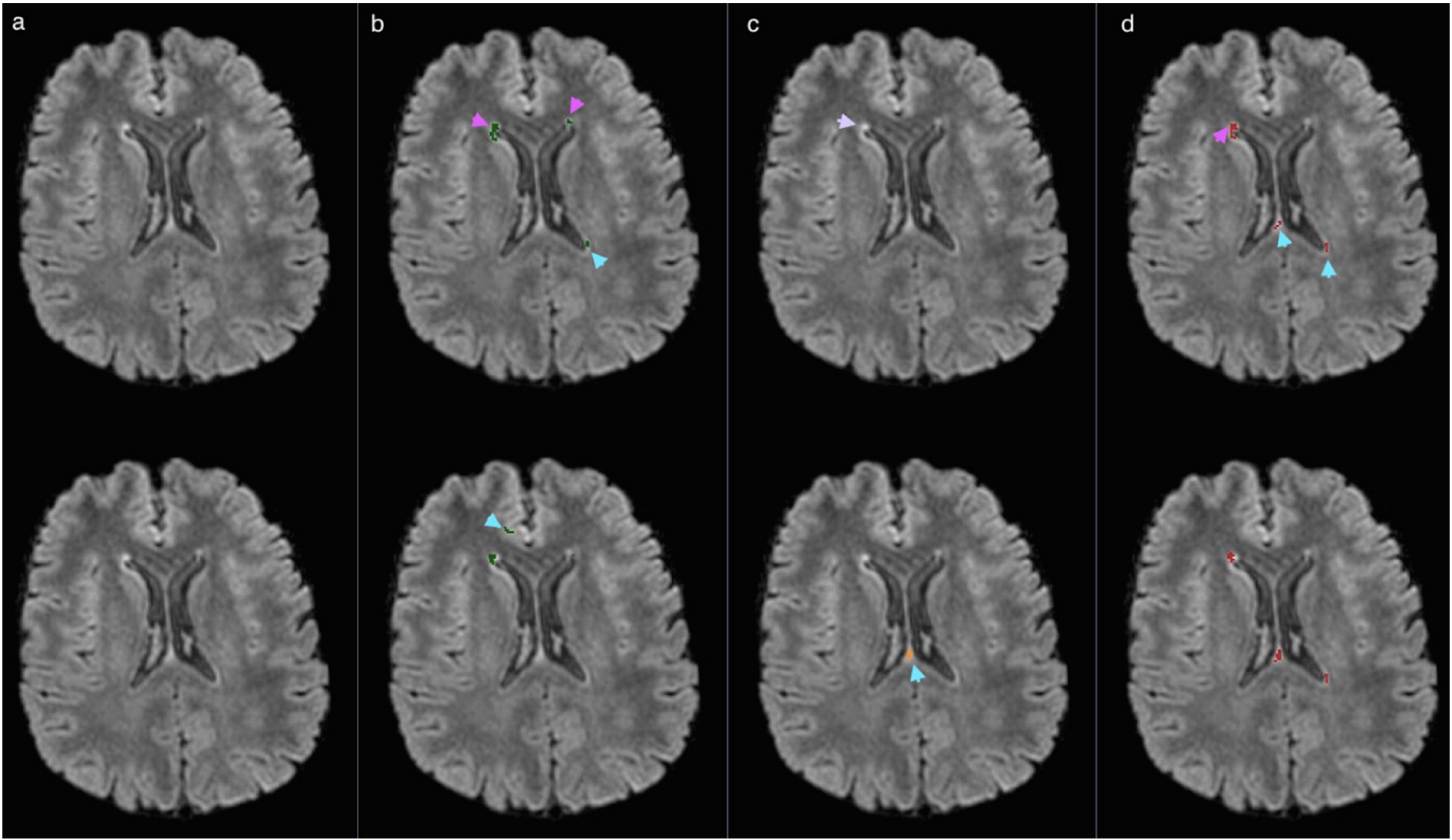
Bias corrected FLAIR image (a) and super-imposed lesion segmentation from: (b) MSmetrix, (c) LST, and (d) Lesion-TOADS. The first row corresponds to the lesion segmentation of scan 1 and the second row corresponds to the lesion segmentation of scan 2. Cyan arrow heads show the difference in the lesion segmentation between scan 1 and scan 2 for MSmetrix, LST and Lesion-TOADS. Pink arrow heads show subtle lesions that are picked up by MSmetrix and Lesion-TOADS. Purple arrow head shows missed subtle lesions by LST.
